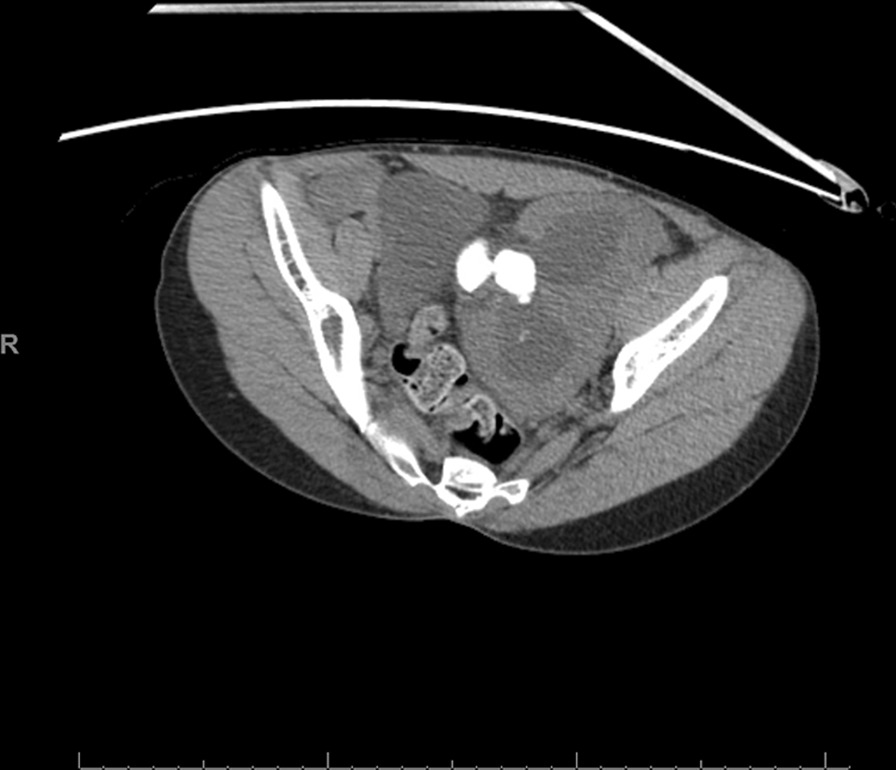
Axial slice of a computed tomography abdomen/pelvis showing a large stone burden in a solitary pelvic kidney
